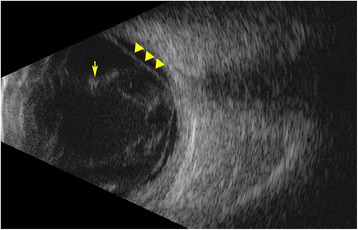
Ultrasound B-scan of a patient diagnosed with endogenous bacterial endophthalmitis following septic arthritis. There is presence of dense, hyper-reflective echoes in the vitreous cavity suggestive of exudates (*yellow arrow*). The membrane-like echo in the scan marked by *yellow triangles* suggests presence of a total retinal detachment
